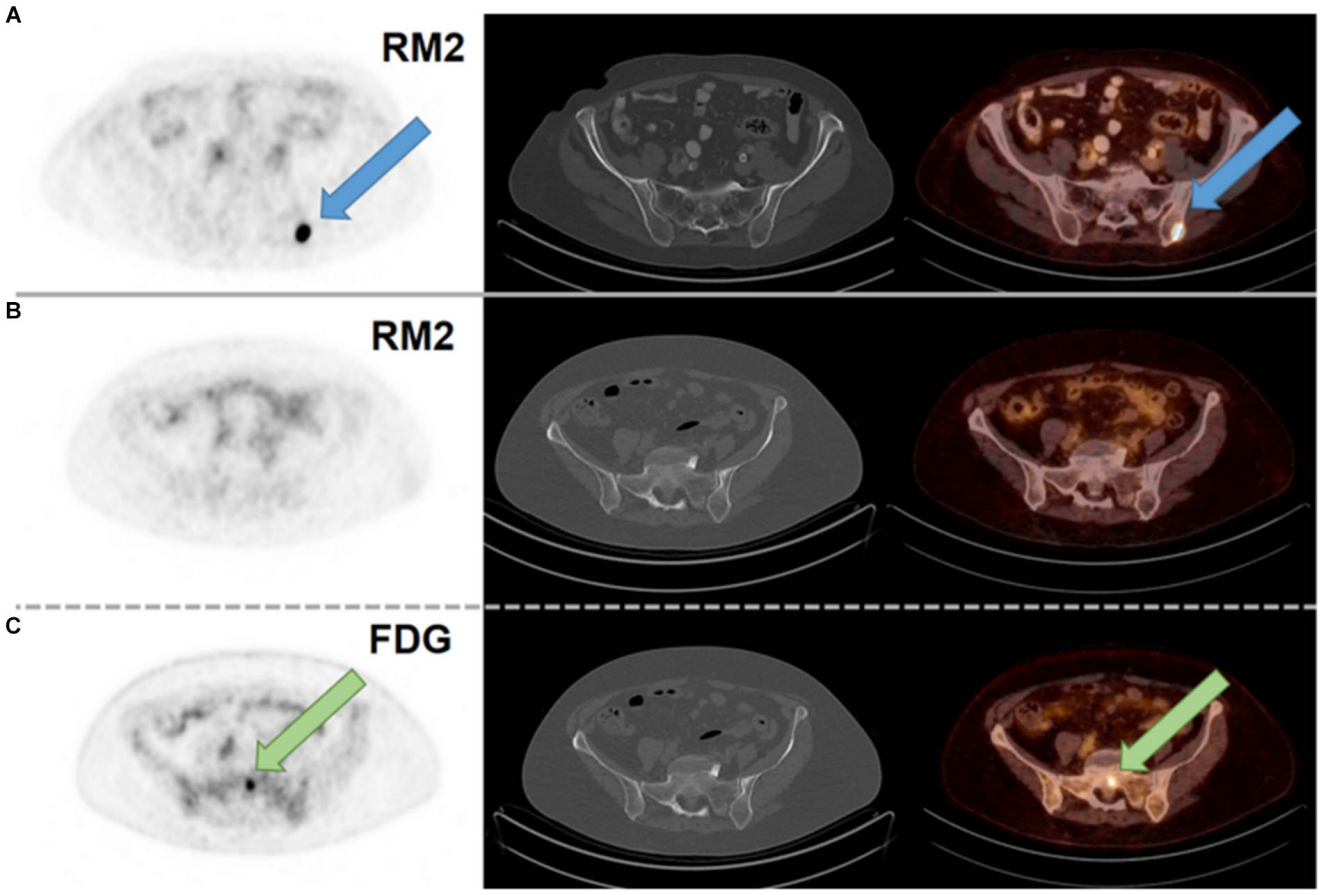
(A) [^68^Ga]Ga-RM2 PET/CT of a patient with a metastasis in the left iliac bone with intense RM2 binding and not seen on CT (blue arrow; SUVmax 32.1). (B) [^68^Ga]Ga-RM2 PET/CT of a patient with a bone metastasis in the sacrum without RM2 binding and not seen on CT (SUVmax 1.5; second row). (C) [^18^F]FDG PET/CT of the same patient with intense hypermetabolism in the sacrum (green arrow; SUVmax 5.8; third row). Axial slices of PET scans (first column), CT scans (second column) and fusion images (third column). Reprinted from Gastrin-Releasing Peptide Receptor Antagonist [68Ga]RM2 PET/CT for Staging of Pre-Treated, Metastasized Breast Cancer by Michalski et al. (102), under Creative Commons Attribution (CC BY) license.
